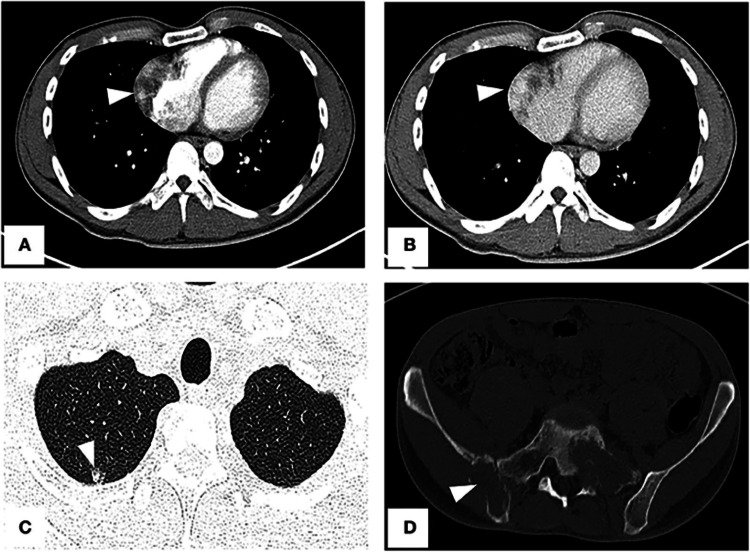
The right atrial tumour, a little ground-glass pulmonary nodule, and bone destructions are seen on an enhanced CT scan of the chest and pelvis. (A) Right atrium filling deficiency is shown on arterial phase imaging (arrowhead). Venous phase imaging (B) reveals the tumour's uneven enhancement (arrowhead). (C) A metastatic nodule can be seen in the upper right lung on a lung window image. (D) Destroyed bones in the sacrum and right ilium. Image Credit: Li et al., 2022 [39]; Licensed under CC-BY
